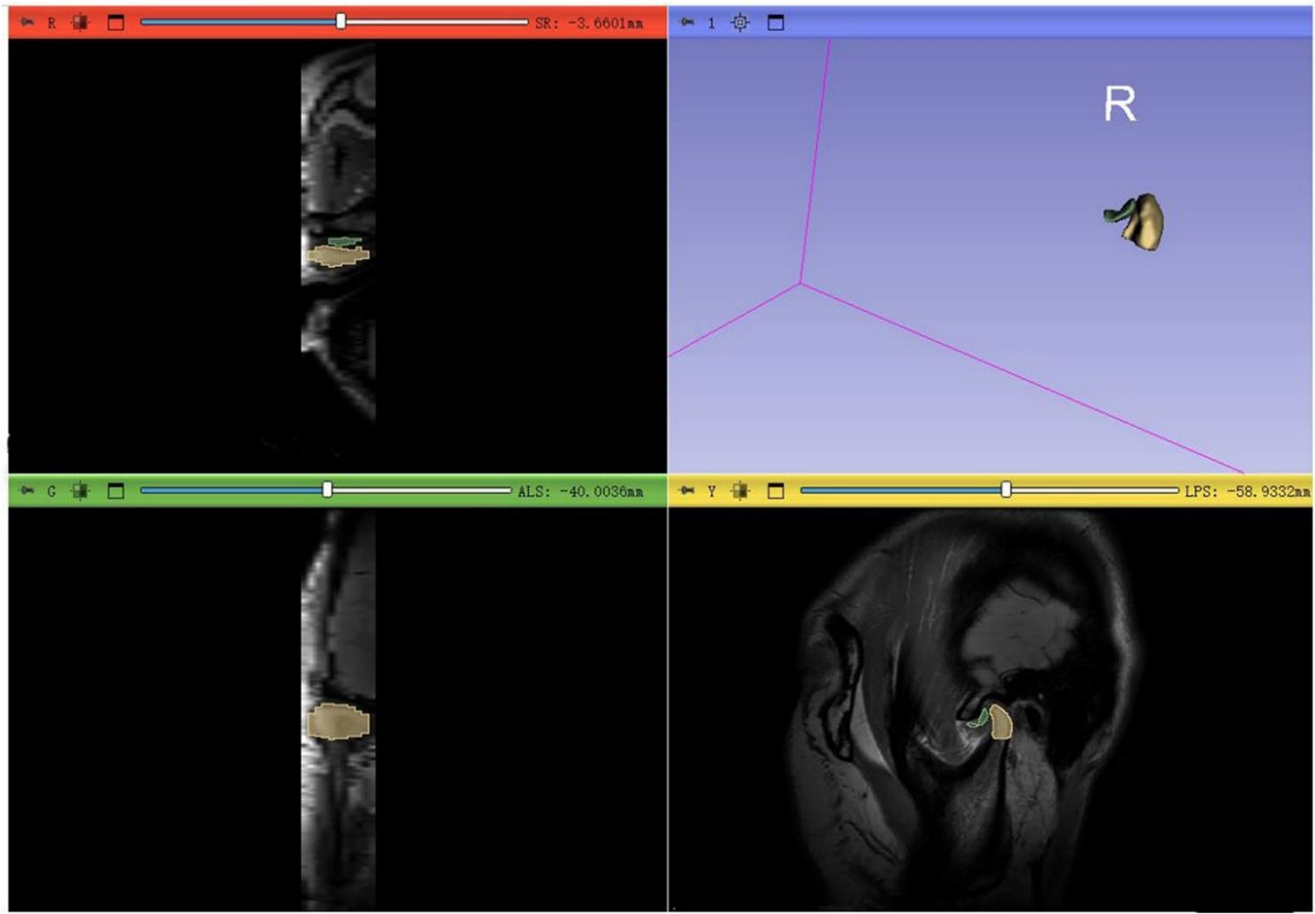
Magnetic resonance imaging (MRI) images showing the tracings of the volume of interest (VOI) in a radiomic (3D-Slice) platform
